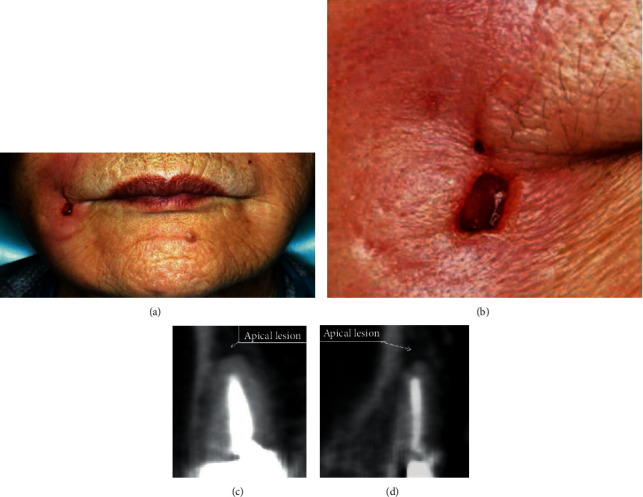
(a) Photograph of the front of the face taken at the first visit. Diffuse swelling of the right cheek was seen. A fistula of about 5 mm was present near the corner of the mouth. (b) Photograph around the fistula. Pus was discharging from the fistula. (c) Cone beamed computed tomography (CBCT) image at the causal tooth in coronal image. (d) CBCT image at the causal tooth in sagittal image. There was an apical lesion of about 3 mm in the root apex of the right maxillary second premolar.
